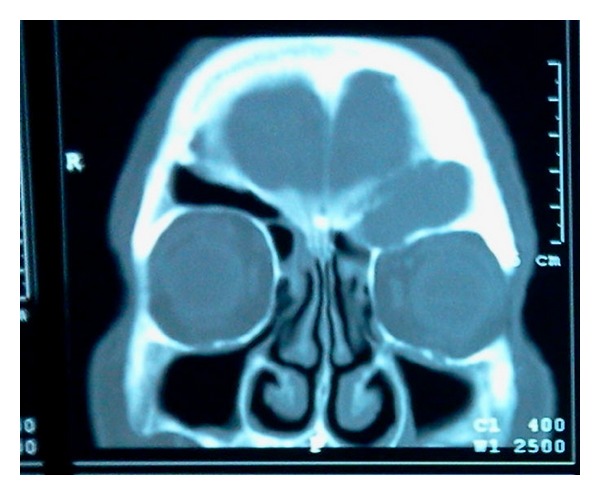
Computed tomography (CT scan) of the orbits shows complete opacity of the left frontal sinus with anterior and inferior breach of the sinus.
